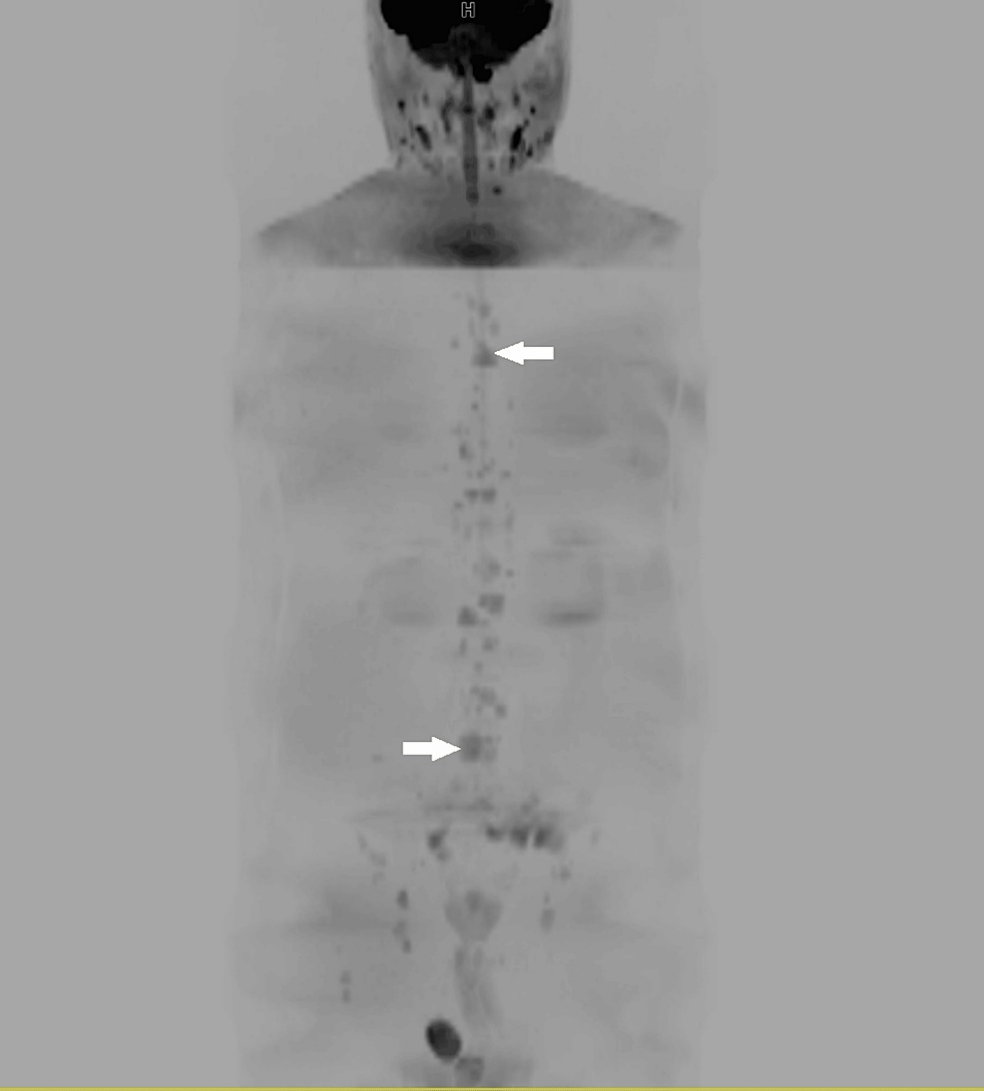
Whole body diffusion-weighted MRI of the same patient with coronal inverted MIP reconstruction showing multiple vertebral metastases (arrows).
